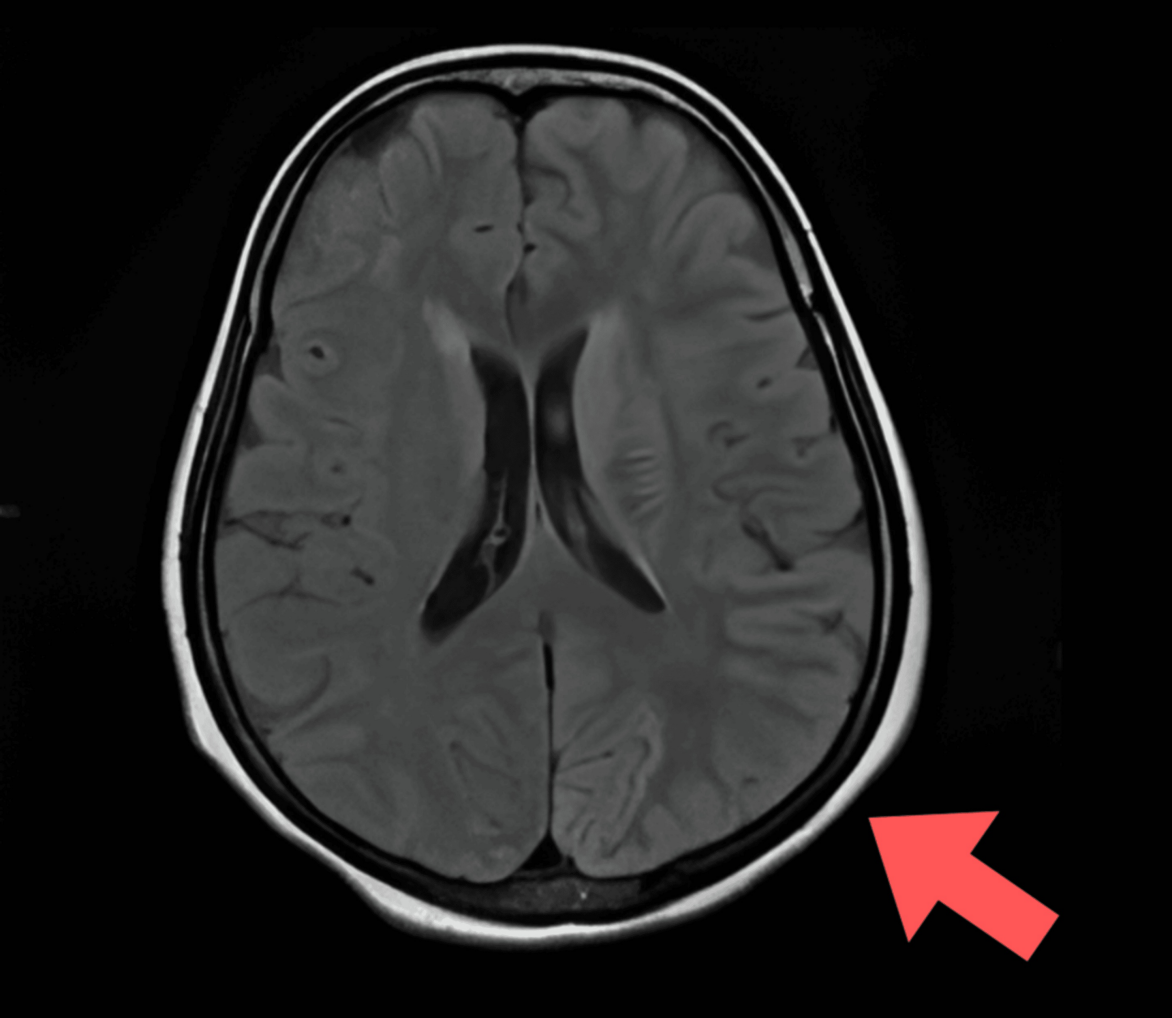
Fluid-attenuated inversion recovery (FLAIR) imaging showing hyperintensity in the right parieto-occipital region.
